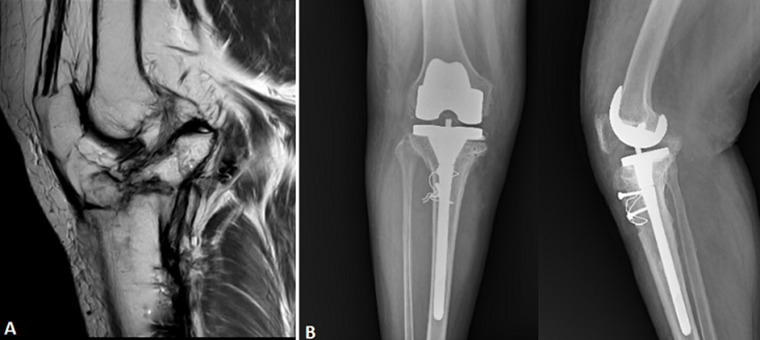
A) magnetic resonance imaging of the right knee demonstrating chronic post-traumatic changes, including thinning of the articular cartilage, degenerative tear of the lateral meniscus, and evidence of an old cleavage fracture involving both tibial plateaus; B) radiographs showing postoperative anteroposterior and lateral views of the right knee showing a well-aligned total knee replacement (TKR) implant with femoral component and tibial stem extension; the presence of cerclage wires and screws indicates prior fixation, consistent with previous management of a complex tibial plateau fracture
